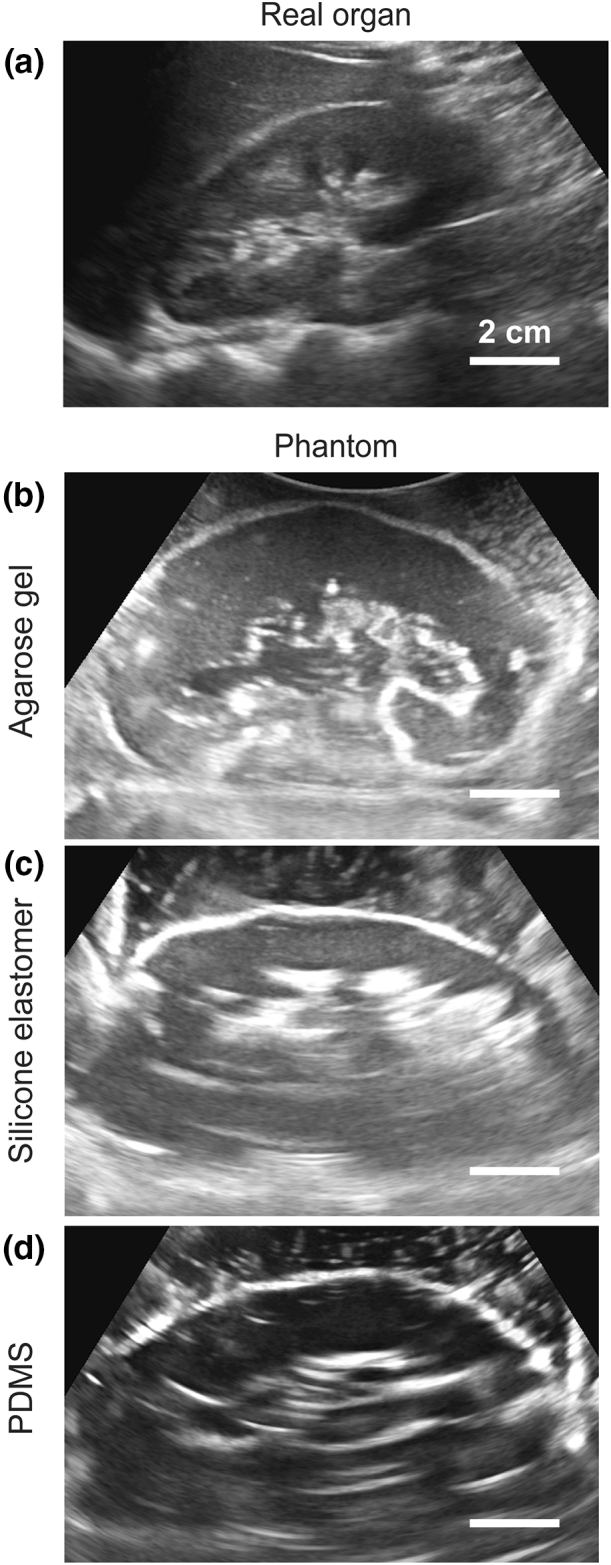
Ultrasound images of the three models made of different materials in comparison to a real human kidney. The agarose model outperforms the other types of materials in terms of replicating the outer shape and tissue of the kidney, especially the appearance of the collection system, when compared to the real organ.
